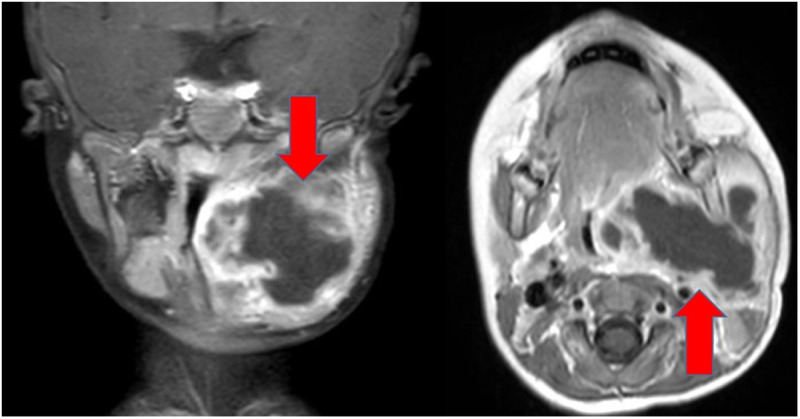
Parapharyngeal abscess in 19-month-old boy. Magnetic resonance imaging (MRI) with intravenous contrast medium of a 19-month-old boy with a parapharyngeal abscess (T1-weighted): The left image shows the coronal plane, the right image the axial plane. The red arrows mark the extensive parapharyngeal abscess on the left side (4.6 x 5.2 x 4.9 cm; cranial to caudal x right to left x ventral to dorsal) with inhomogeneous diffusion disturbance and marginal accumulation of contrast medium. The abscess extends around the mandible, is not directly related to the internal carotid artery and surrounds the external carotid artery. It extends cranially to the nasopharynx and reaches the thyroid gland caudally. The abscess formation displaces the oropharyngeal, hypopharyngeal and cervical structures to the opposite side, especially the trachea. The adjacent soft tissue structures show inflammatory edematous changes.
